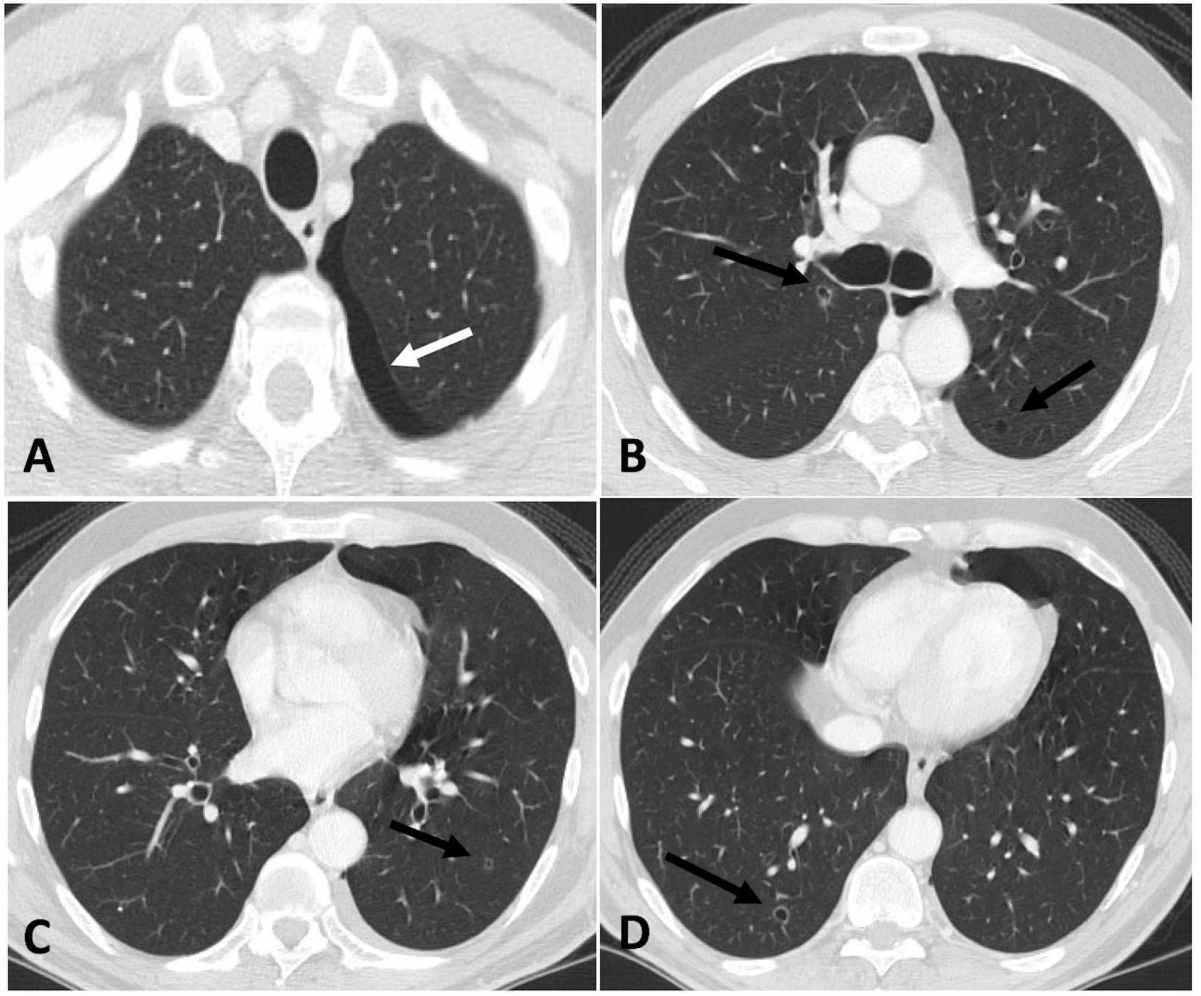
Enhanced CT at three-month follow-up (lung window) The images show multiple round thin wall cysts (black arrows in B, C, and D) and left pneumothorax (white arrow in A) CT: computed tomography
